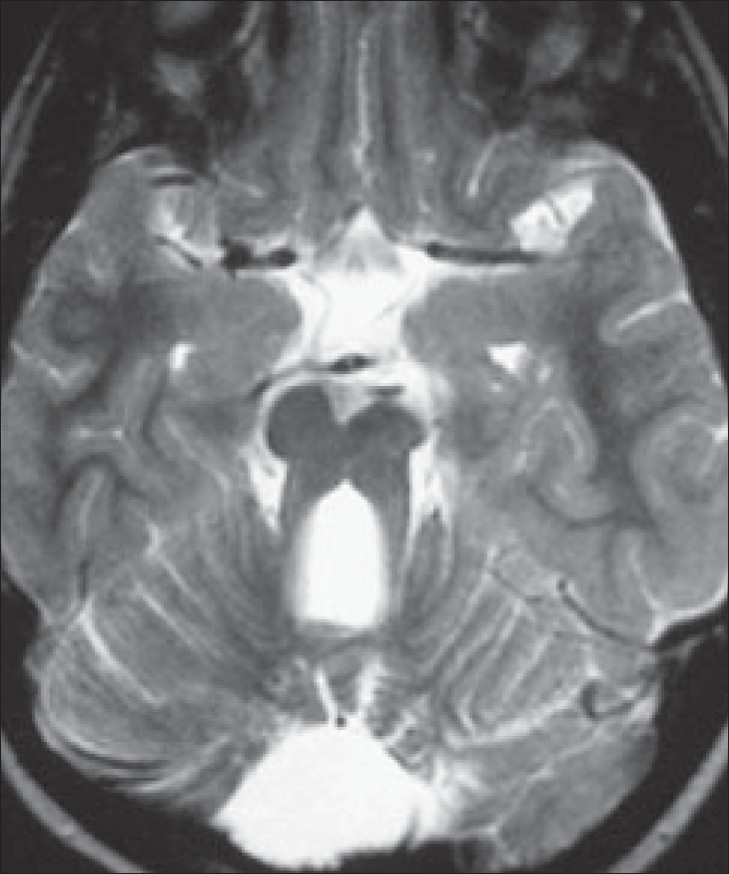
Axial T2W MRI of the brain
